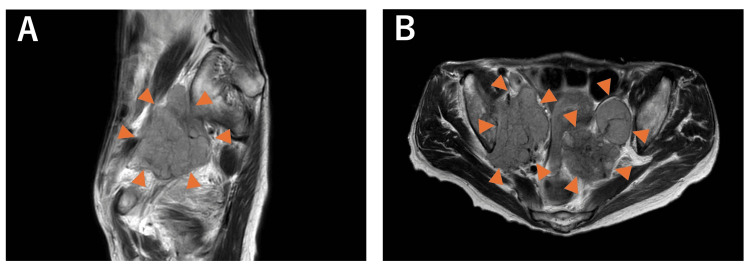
Pelvic contrast-enhanced MRI of case one Axial (A) and sagittal (B) T2WI images revealed a mass with intermediate signal intensity.
